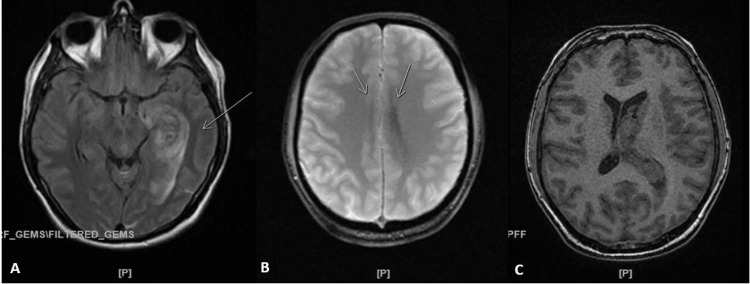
Mri of the brain demonstrating the extension of intraventricular hemorrhage, midline shift, and bilateral subarachnoid hemorrhages A multiplanar/multi-sequence MRI of the brain was performed without contrast. A: FLAIR, axial view. Seen is a re-demonstration of left deep temporal lobe hemorrhage (arrow) extending into the left lateral ventricle and third ventricle B: GRE image, axial view. Arrows indicate bilateral subarachnoid hemorrhage involving both hemispheres up to the high convexity C: T1 axial 3D image. Observed is a midline shift measuring approximately 6 mm FLAIR: Fluid-attenuated inversion recovery, GRE: Gradient echo
